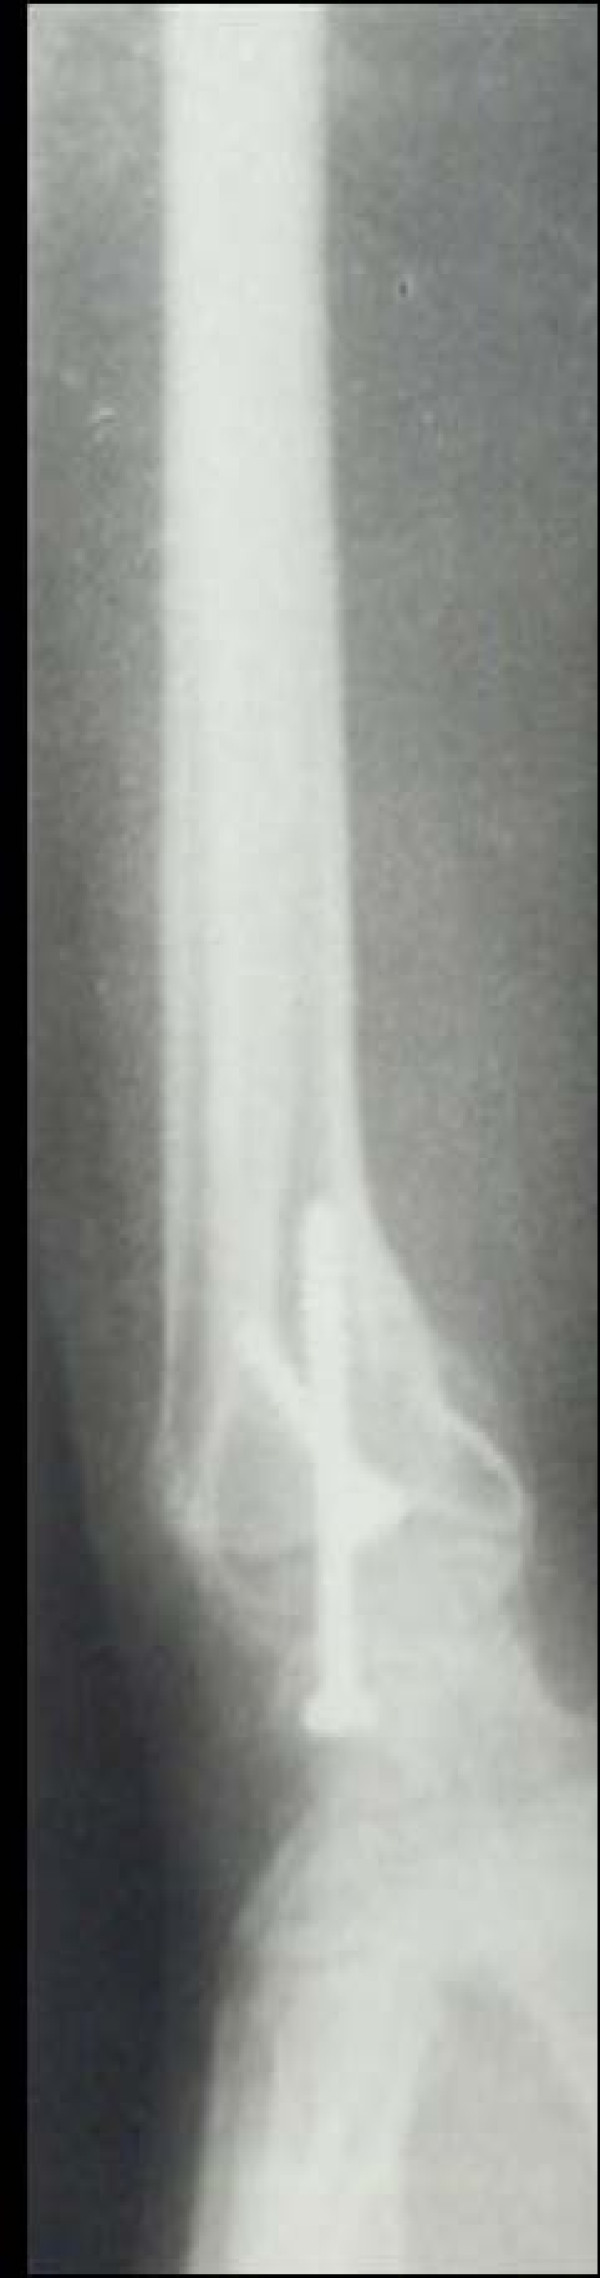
Case 1 Radiograph (Lateral view) at 7 year followup.
